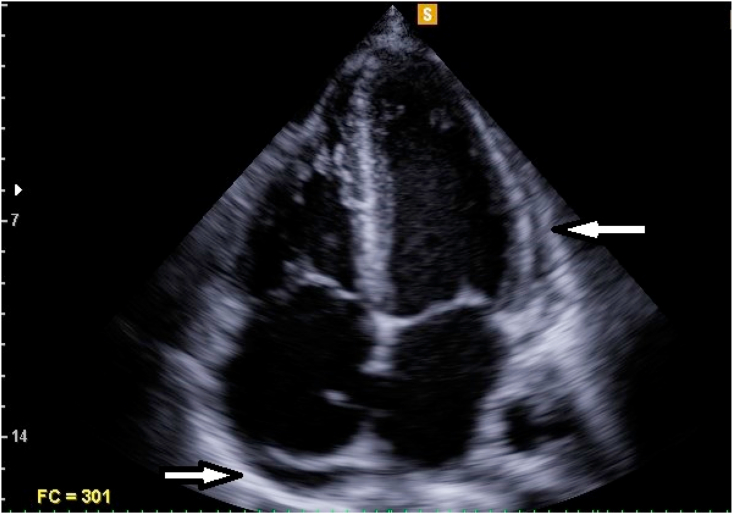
The small pericardial effusion in the first echocardiography.
